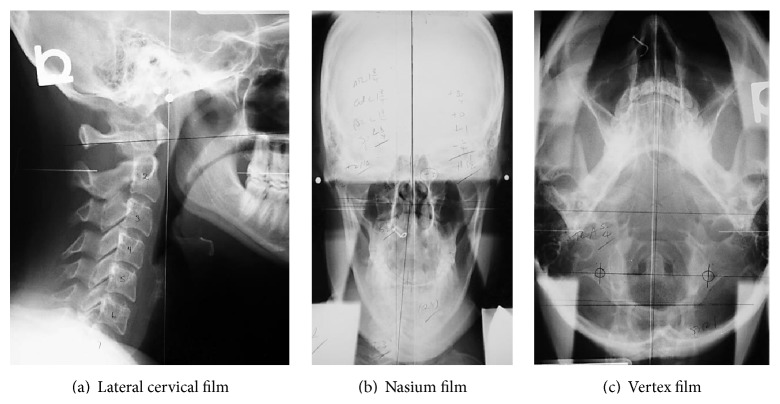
NUCCA radiograph series. These films are used to determine atlas misalignment and developing a correction strategy. After-correction radiographs or postfilms ensure the best correction has been made for that subject.
